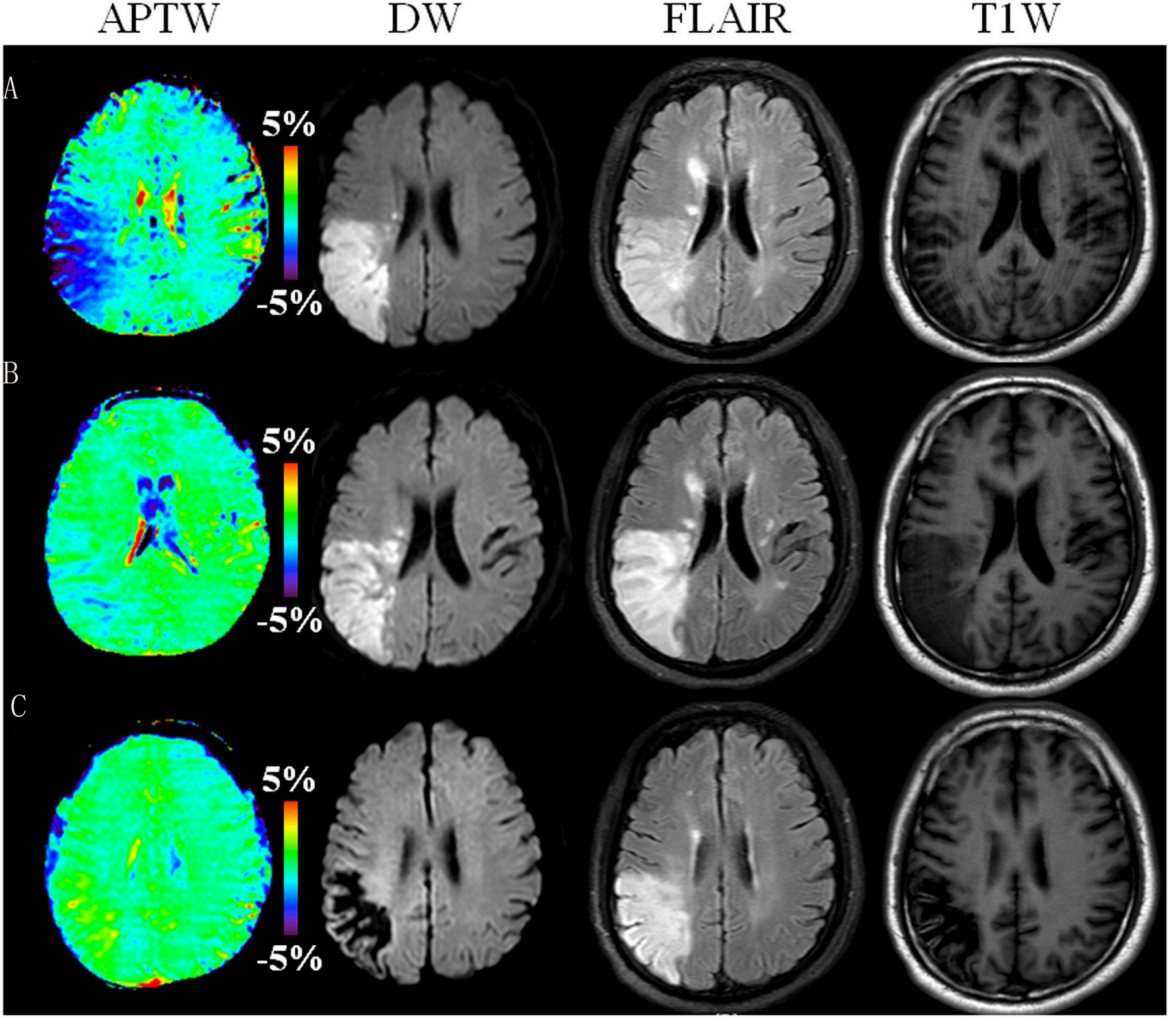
APTW, DW, FLAIR, and T_1_-weighted (T_1_W) images of a representative patient with ischemic stroke (male, 52 years old) at 1 day (A), 6 days (B), and 34 days (C) post-onset (or pre-treatment, 5 days, and 33 days post-treatment). DW images showed a hyperintensity in the lesion on the first and second scans, but hypointensity on the third scan. APTW images showed gradually increased signal intensity values in the corresponding lesion area. The NIHSS was 6, 3, and 2 at 1, 6, and 34 days post-onset, respectively. Note that the geometry and location of the slice was not positioned very well at the last time point.
